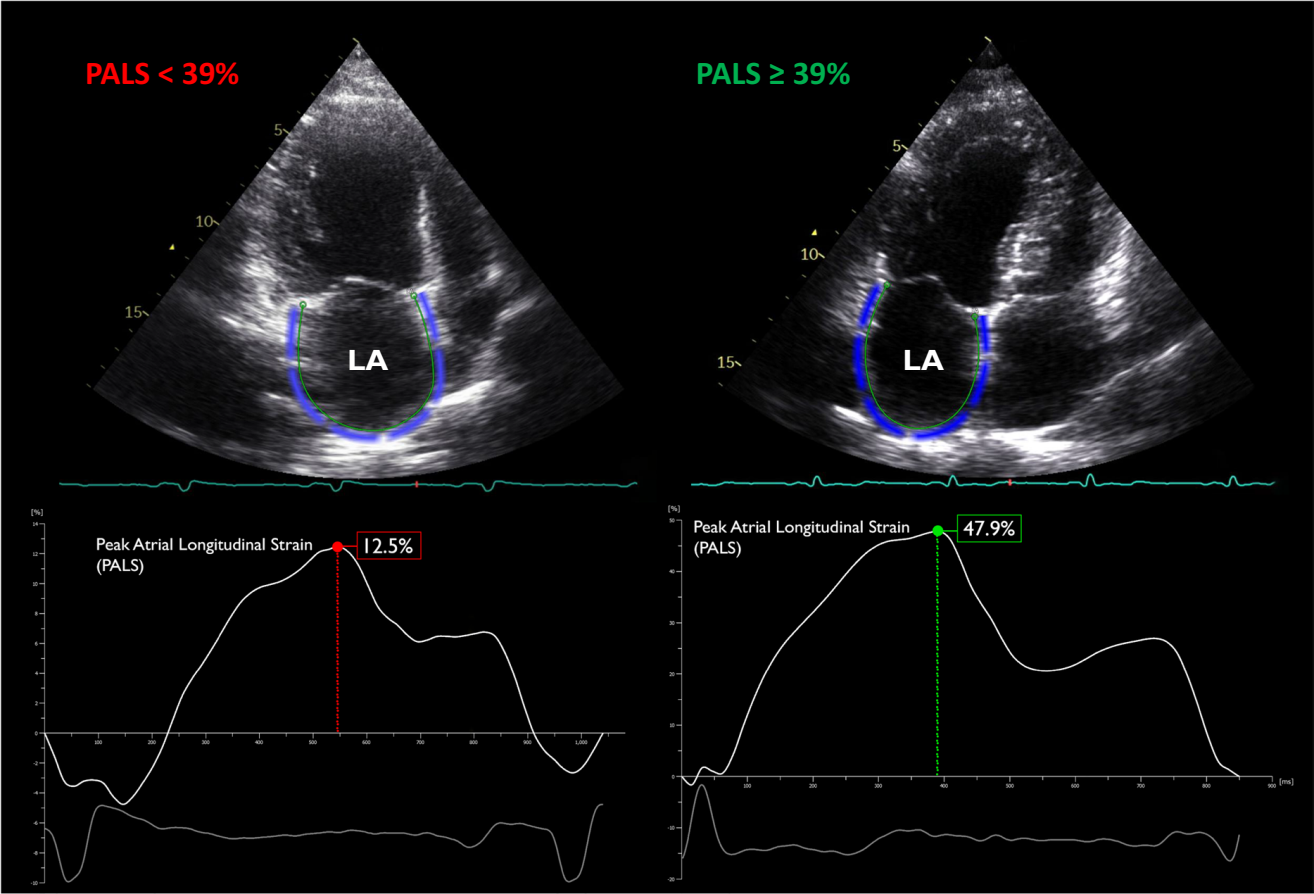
Representative cases of subjects below (indicated with red) and above 39% (indicated with green) of peak atrial longitudinal strain (PALS). Subject with 12.6% PALS met the primary endpoint during the follow-up period. The blue contour on the 2D echocardiographic image depicts left atrial (LA) endocardial border at end-systole
